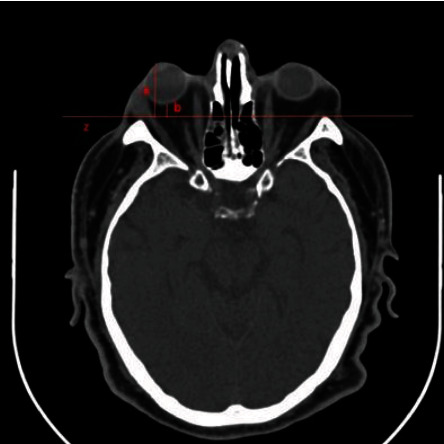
Axial CT brain at the level of the optic nerve head. Note the prominence of retroorbital fat. z: interzygomatic line; a: distance to the anterior sclera is 29.9 mm; b: posterior sclera is 6.3 mm anterior to the interzygomatic line.
